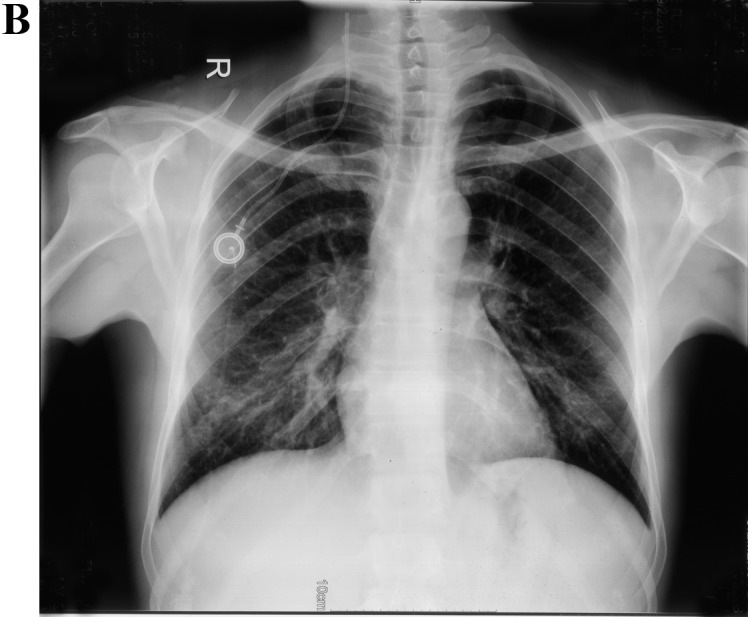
A 36-year-old male patient with colon carcinoma. (A) Upright posteroanterior chest radiograph showing that a right subclavian port was implanted into the chest wall and that the catheter was placed into the lower half of the vena cava just after the procedure. (B) Malposition due to dislocation of this port catheter can be observed in the right jugular vein. This developed 3 months later. The catheter was subsequently placed back into the vena cava via jugular access.
